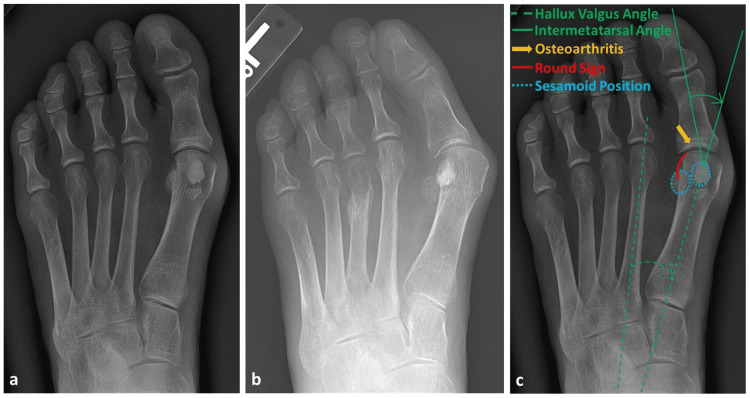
Radiographs of a 54-year-old female patient (a) An initial encounter radiograph and (b) an 85-month follow-up radiograph. Increases in HVA, IMA, osteoarthritis, Hardy-Clapham sesamoid position, and round sign are observed. Labeled measures (c) are provided in the initial encounter radiograph.
